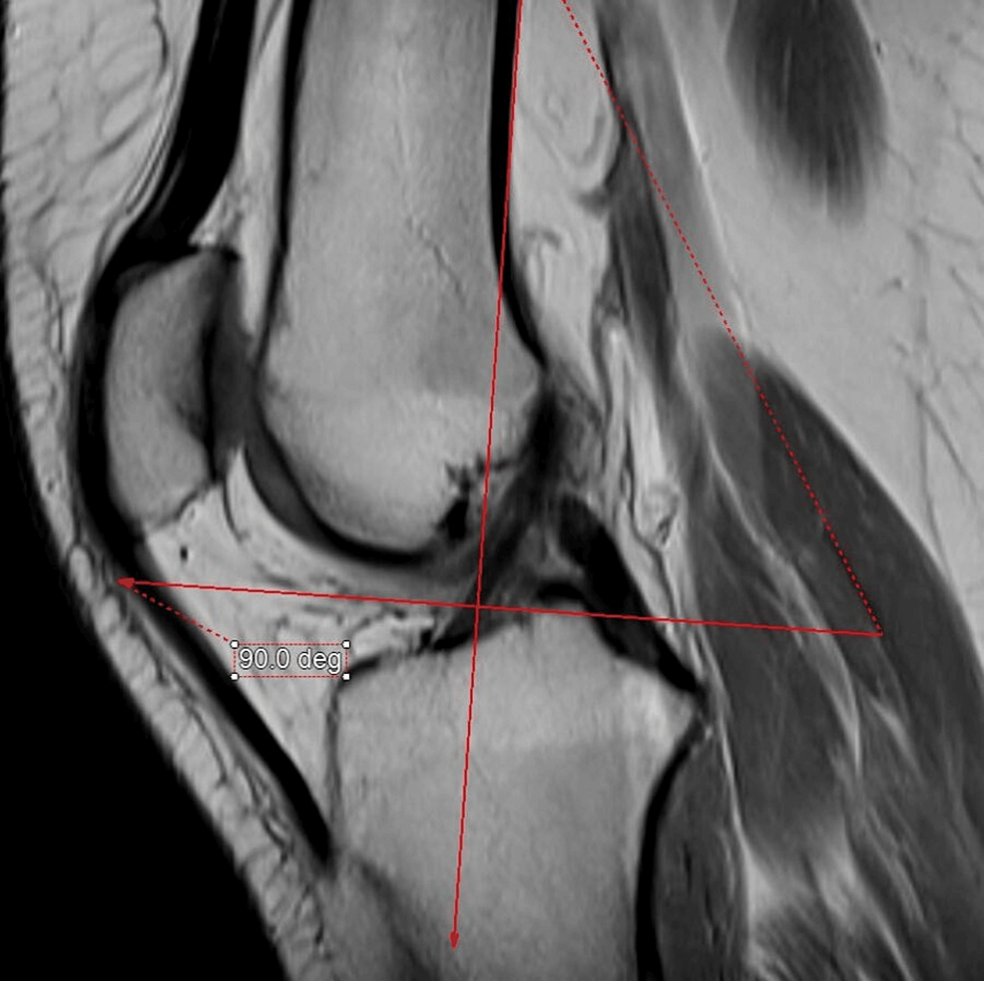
Sagittal proton-density MRI of the knee at the midsagittal plane. Sagittal proton-density MRI of the knee. The femoral posterior cortical axis was identified and drawn in the midsagittal plane.
